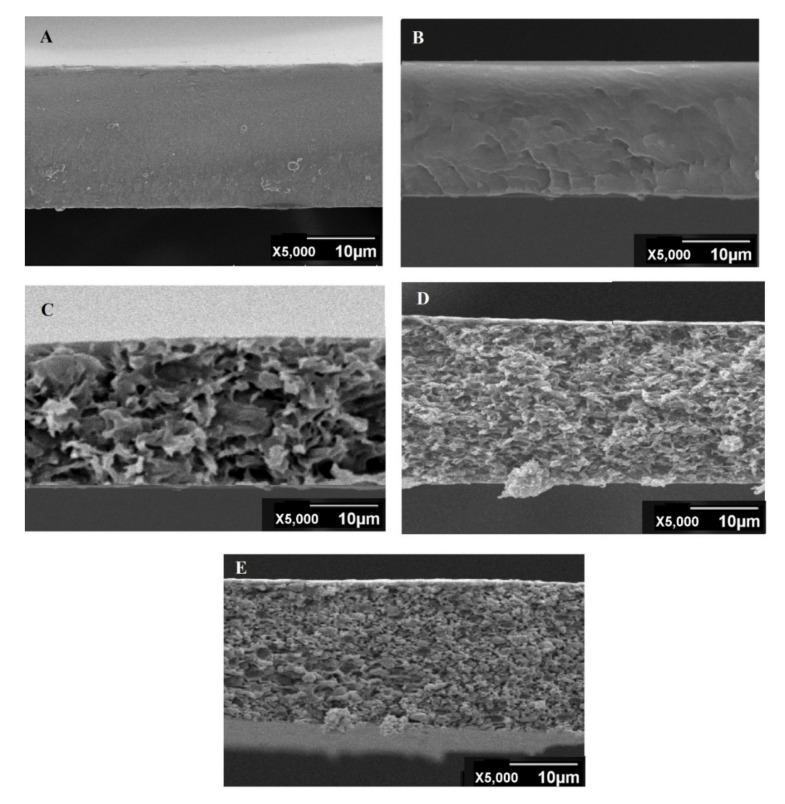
Cross-section SEM images of (A) CTA:CDA (100:0), (B) CTA:CDA (0:100), (C) CTA:CDA (80:20), (D) CTA:CDA (60:40) and (E) CTA:CDA (50:50).
